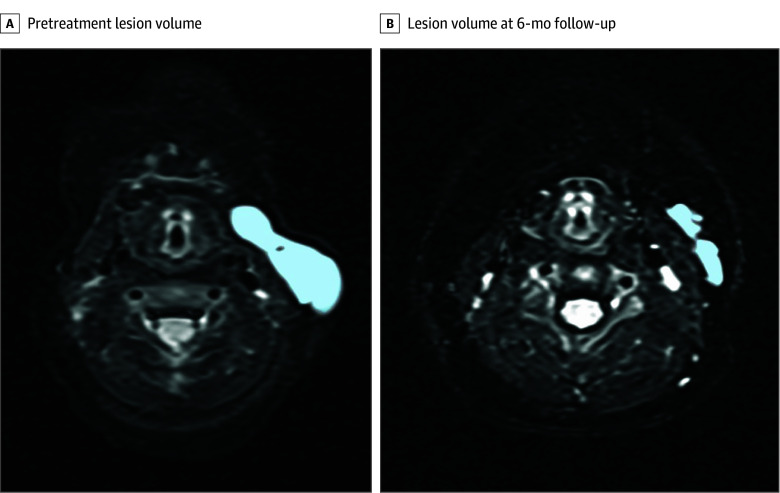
Magnetic Resonance Imaging (MRI)–Based Volumetric Analysis of a Representative Patient MRI (T2-weighted) scan of a 1-month-old infant with a lymphatic malformation on the anterior surface of the sternocleidomastoid muscle. A, Pretreatment MRI scan showing a cystic lesion with a volume of 4.71 cm^3^. B, MRI performed at 6-month follow-up after initiating eppikajutsuto revealed a marked reduction, with a final lesion volume of 0.19 cm^3^. Volumetric measurements were obtained using an OsiriX DICOM viewer.
